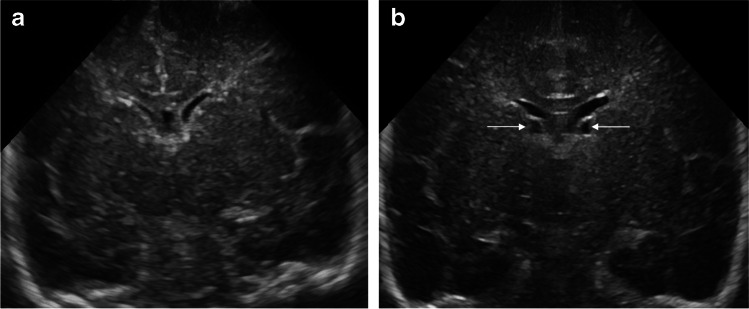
A coronal image from a normal head ultrasound (US) at 7 days of life in a boy born at 29 weeks’ gestational age (a). A coronal image from a follow-up US at 29 days shows new bilateral posthemorrhagic cysts (*arrows*) compatible with prior grade 1 germinal matrix hemorrhage (b)
